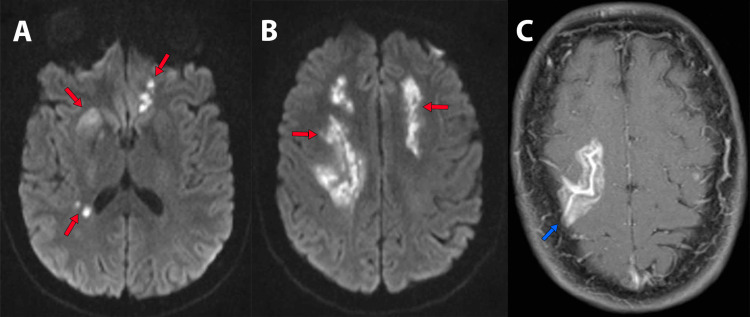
A-B, MRI-DWI shows bilateral white matter hyperintensities at centrum semiovale and high convexity level affecting the ACA-MCA watershed territories (red arrows). C, T1 postcontrast MRI showed leptomeningeal enhancement along the right cortical sulci compatible with an “ivy sign” (blue arrow). DWI: diffusion-weighted imaging, ACA: anterior cerebral artery, MCA: middle cerebral artery
